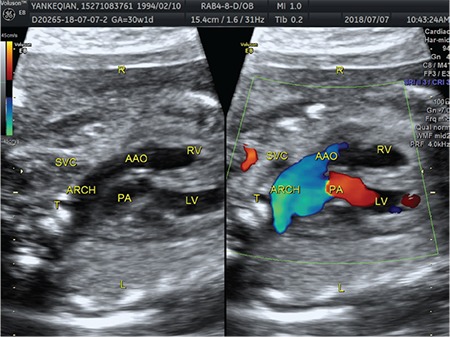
Prenatal ultrasound of a female, 30 + 1 weeks of gestation, showing TGA. Prenatal echocardiography exhibited parallel initial segments of the aorta Pas. AAO: ascending aorta; ARCH: aortic arch; LV: left ventricle; PA: pulmonary artery; RAA: right atrial appendage; RV: right ventricle; SVC: superior vena cava; T: trachea; TGA: transposition of the great arteries
